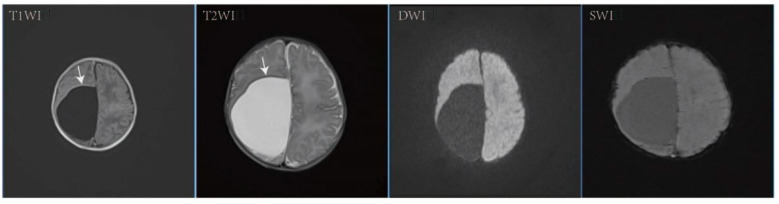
MRI images of a large parieto-occipital cyst.
